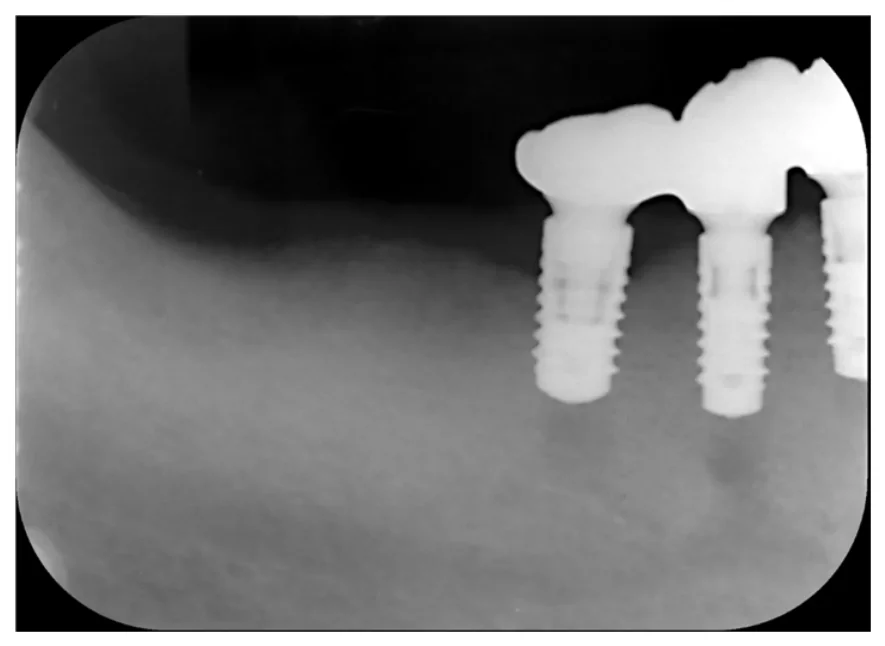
Periapical radiograph of the right posterior region obtained seven weeks after delivery of the definitive prosthesis (29 July 2026). The distance from the implant platform to the crestal bone was greater at #46 than at #44 and #45.
